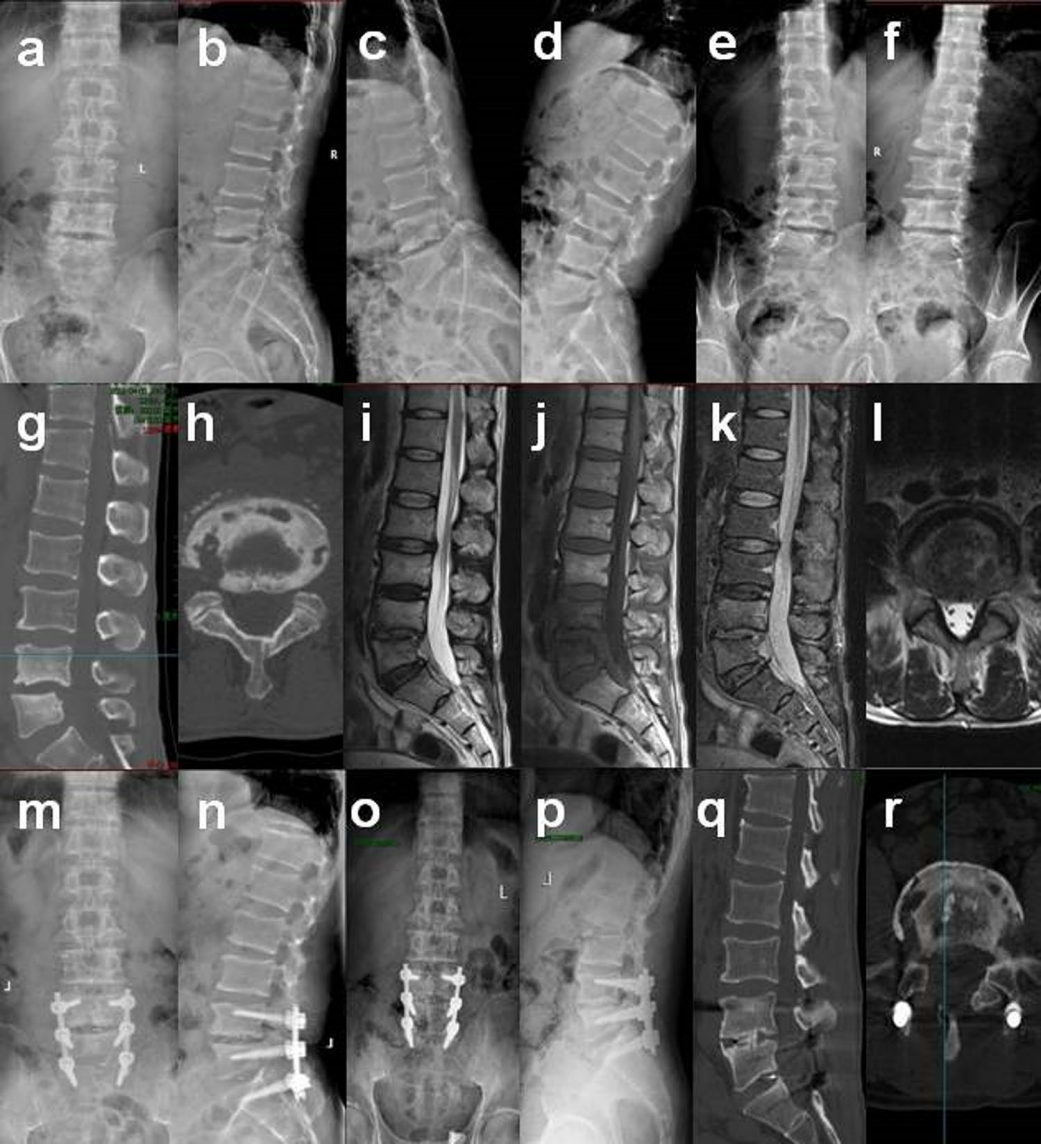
Preoperative and postoperative imaging findings of typical patients. (a–f) Preoperative X-ray showed L4 vertebral body posterior slippage and lumbar instability in a dynamic position, and no obvious isthmus was found in a double oblique position. (g,h) Preoperative CT showed L4–5, L5–S1 multiple lesions at the upper and lower edges of the vertebral body, especially L4–5. (i–l) Preoperative MRI showed L4–5 and L5–S1 uneven abnormal signal changes in the vertebral body and intervertebral disc. (m,n) Two weeks after the operation, X-ray showed L4 spondylolisthesis reduction. (o,p) One year after the operation, the X-ray showed no apparent loss of spondylolisthesis reduction, and the spine was stable. (q,r) One year after the operation, CT showed interbody fusion had been achieved, and the damaged area was repaired well.
